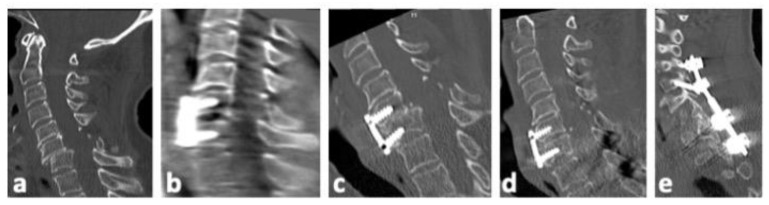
Representative CT images of patient no. 6 (Table 6). (a). Postinjury midline sagittal CT reconstruction. (b). Intraoperative 3D scan after anterior open reduction, discectomy, and fixation with intervertebral allogenous bone graft and plate C6/7. (c). Secondary dislocation of C6/7 nine days post operation. (d,e). Additional posterior reduction and stabilization of C4/5 to Th1/2 was performed.
